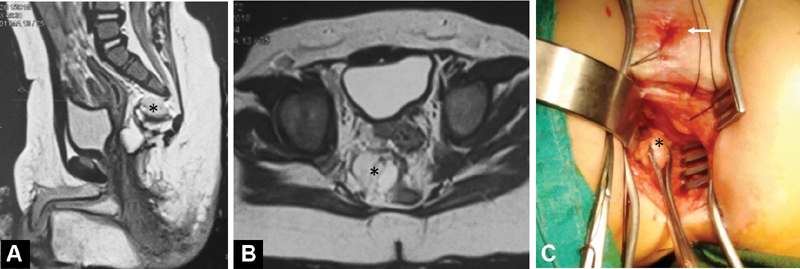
Follow-up magnetic resonance imaging (MRI) study performed after posterior sagittal anorectoplasty showing a residual presacral mass (*) in midsagittal T2-weighted image (
